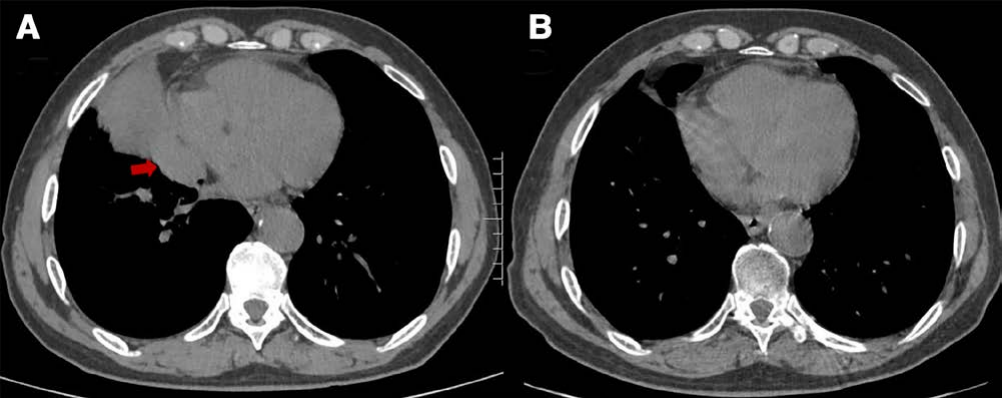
(A) CT scan on July 2, 2022, showing a mass of soft tissue in the middle lobe of the right lung (indicated by the red arrow) with bronchial obstruction and atelectasis. (B) Follow-up CT scan on November 6, 2024, demonstrating significant shrinkage of the right middle lobe mass after treatment, consistent with favorable therapeutic response and accompanied by marked relief of cough and sputum symptoms. CT = computed tomography.
